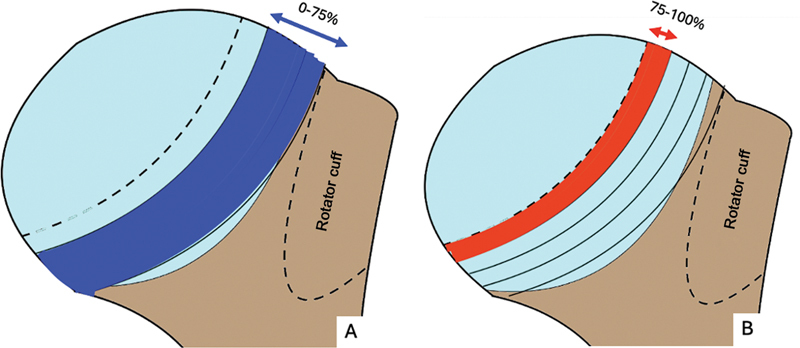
Magnetic resonance imaging scan evaluated in the axial section (black arrow); three-dimensional CT scan evaluated perpendicularly to the defect (blue arrow). Note that the black arrow is larger than the blue arrow, which may overestimate the defect and change the case management.
